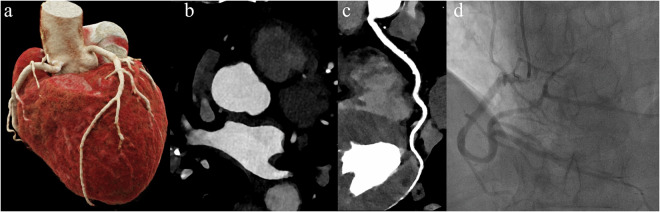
CCTA in a 64-year-old man (HR = 86 bpm, BMI = 26.3 kg/m^2^, Contrast agent flow = 1.8 mL/s, contrast agent volume = 20 mL, CAD-RADS = 1) with chest pain for 3 days. a shows the heart cine volume rendering technology images. b showed CT value of ascending aorta was 370 HU. c, d Representative curved multiplanar reformation of the right coronary artery, along with percutaneous coronary angiography, reveals mild stenosis in the mid-segment
